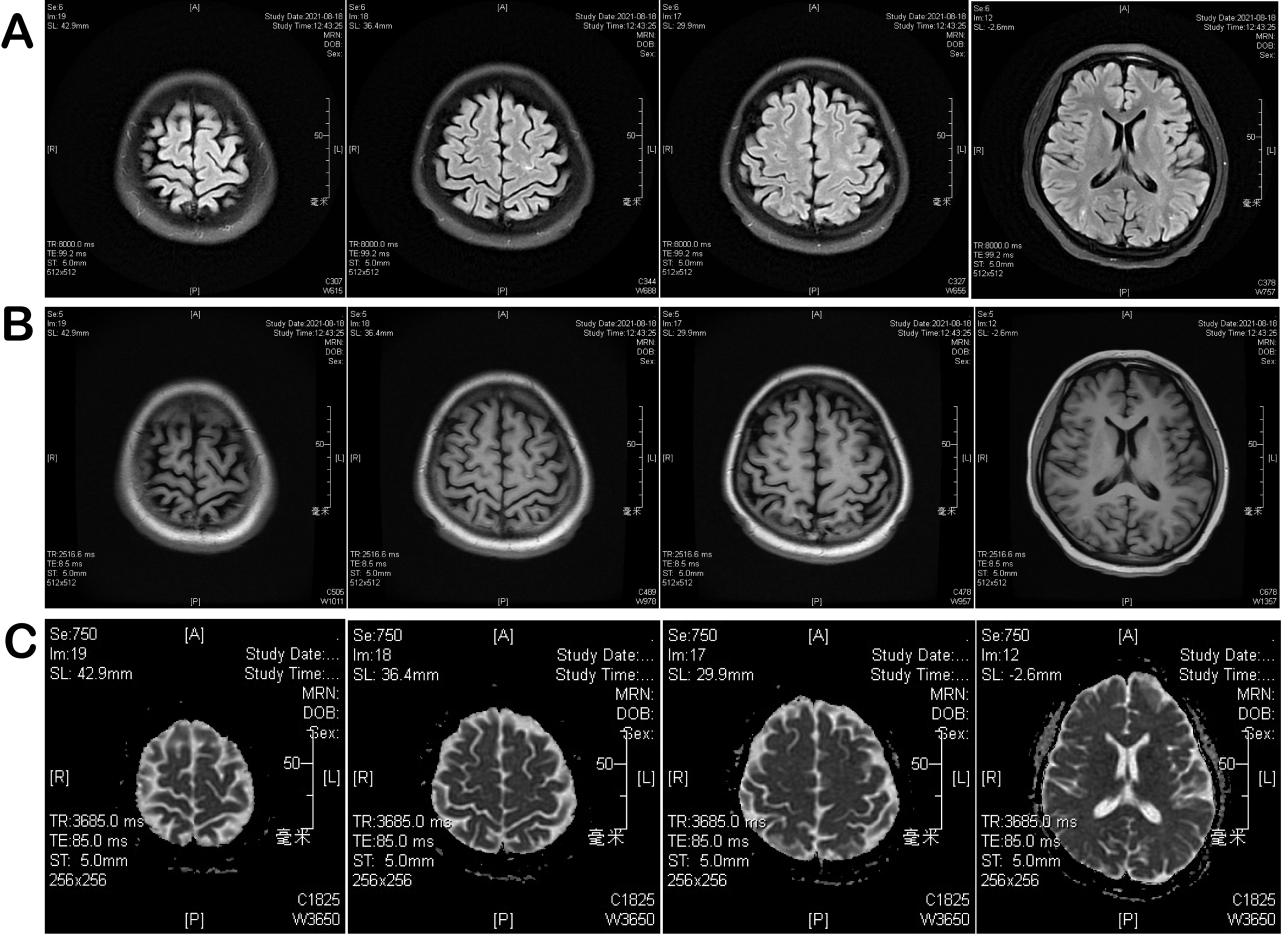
MRI performed on August 14, 2022: (A) Brain/spinal fluid-attenuated inversion recovery (FLAIR) sequence; (B) T1-weighted gadolinium-enhanced sequence; (C) diffusion-weighted imaging and apparent diffusion coefficient (DWI-ADC) maps.
